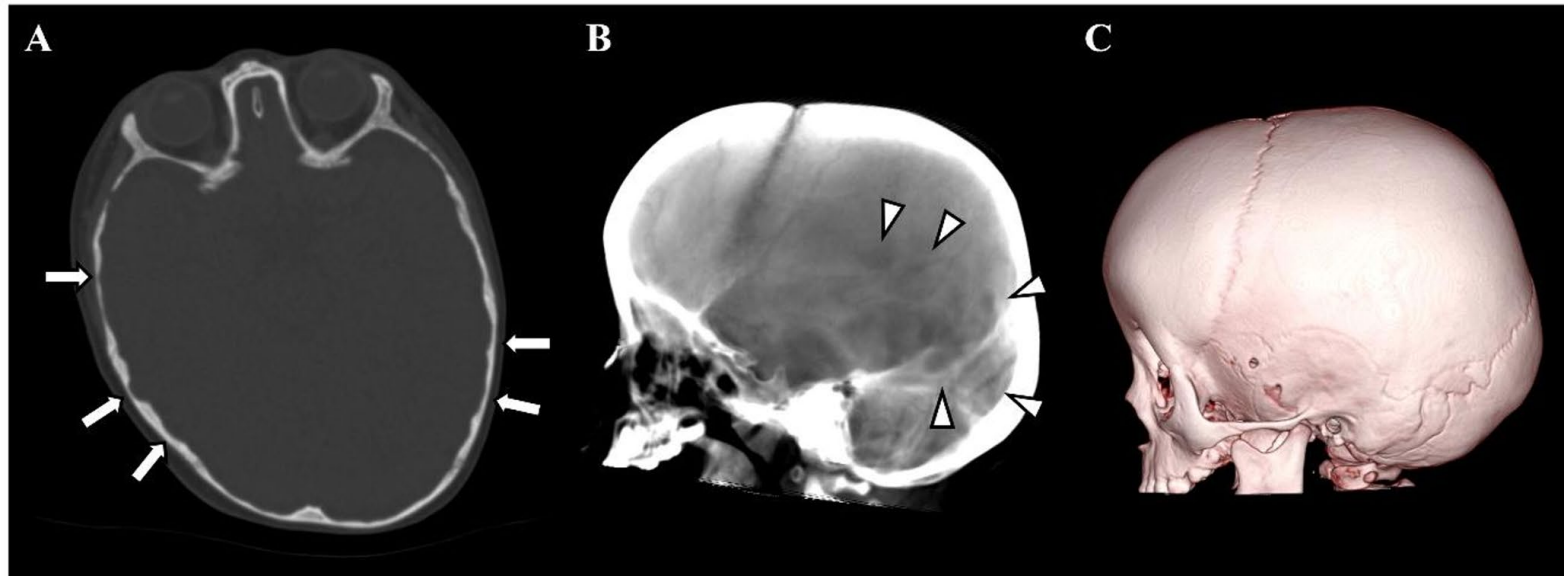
A 22-month-old boy with occipito-frontal circumference, enlarged, who did not show ICH. A Axial head CT image demonstrating multiple CT digital markings at bilateral temporal and parietal bones (arrows). B Ray-sum image demonstrates multiple focal hyperlucent areas due to CT digital markings at posterior side of parietal bone and occipital bone (arrowheads). C VR image demonstrating no focal decompression or holes. This may have been due to the external table of cranial bone growing and being thick enough to be recognized by the image reconstruction software: VINCENT
